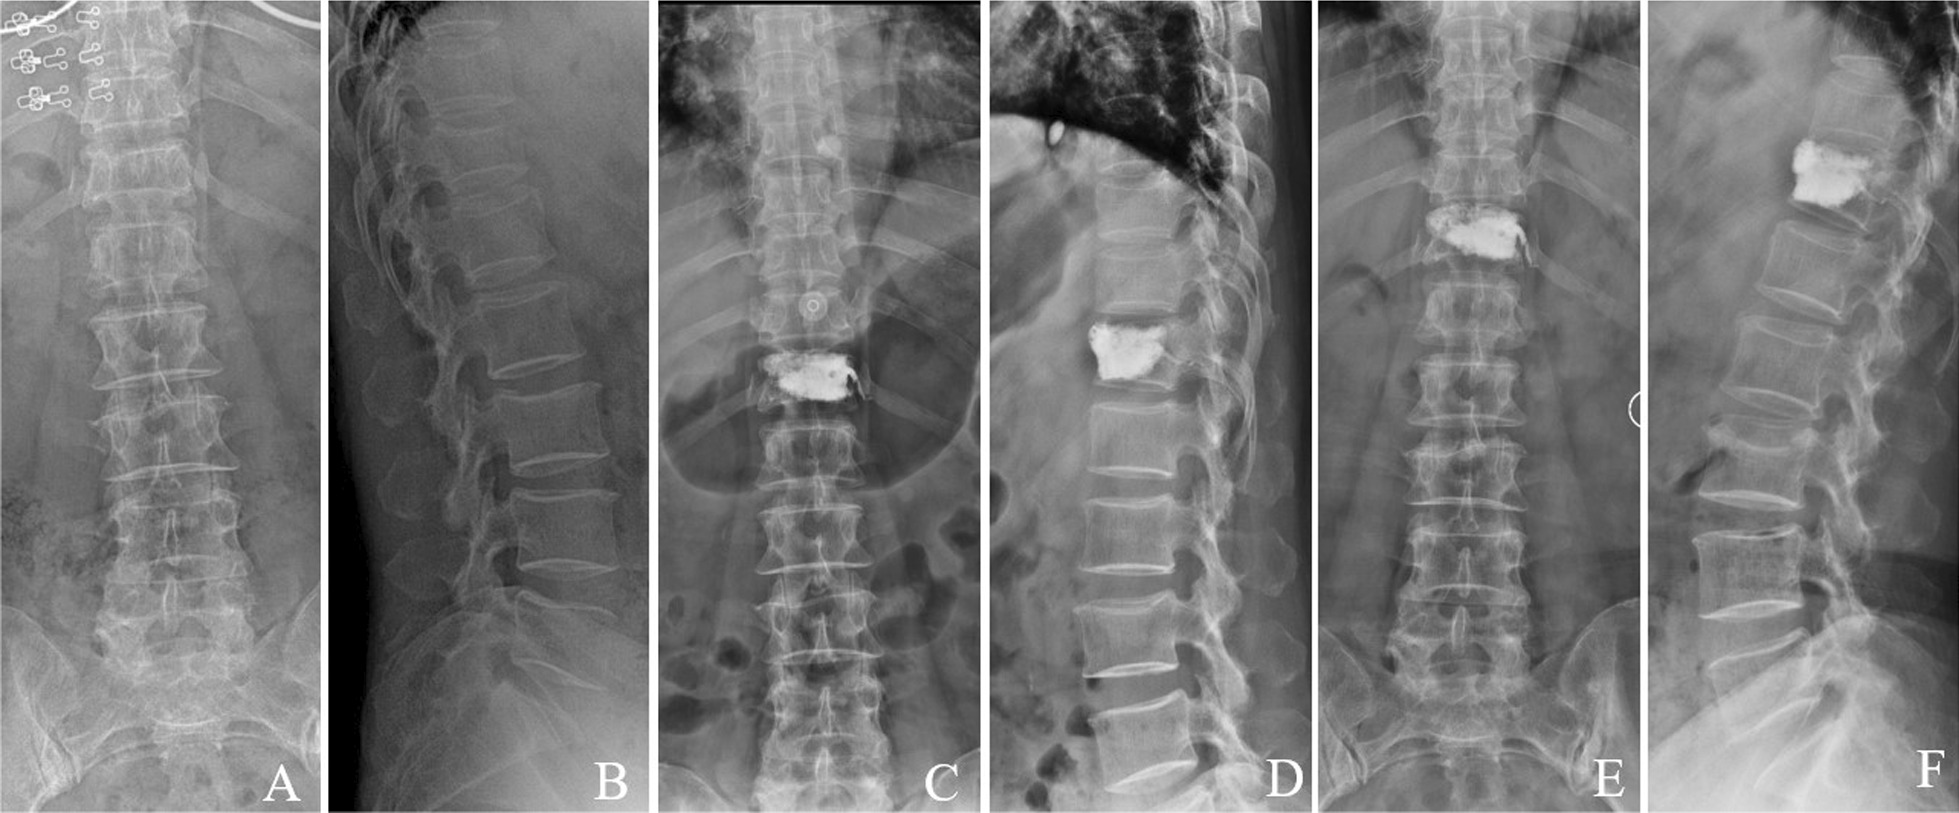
A 77-year-old female with single-level T_12_ OVCF treated by unilateral PKP with directional bone cement delivery device. A, B Preoperative anteroposterior and lateral radiographs showed a T_12_ fracture. C, D, one week after operation, the symmetrical distribution of bone cement. E, F, 12 months after operation: the bone cement remains symmetrically distributed. The anterior height of the injured vertebra and the kyphotic angle has not been changed
